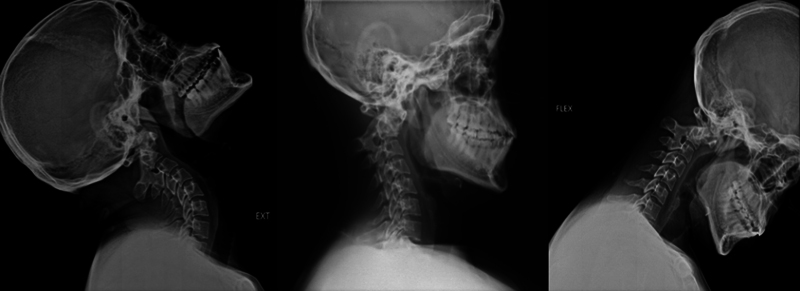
Dynamic radiographs of sagittal planes.
